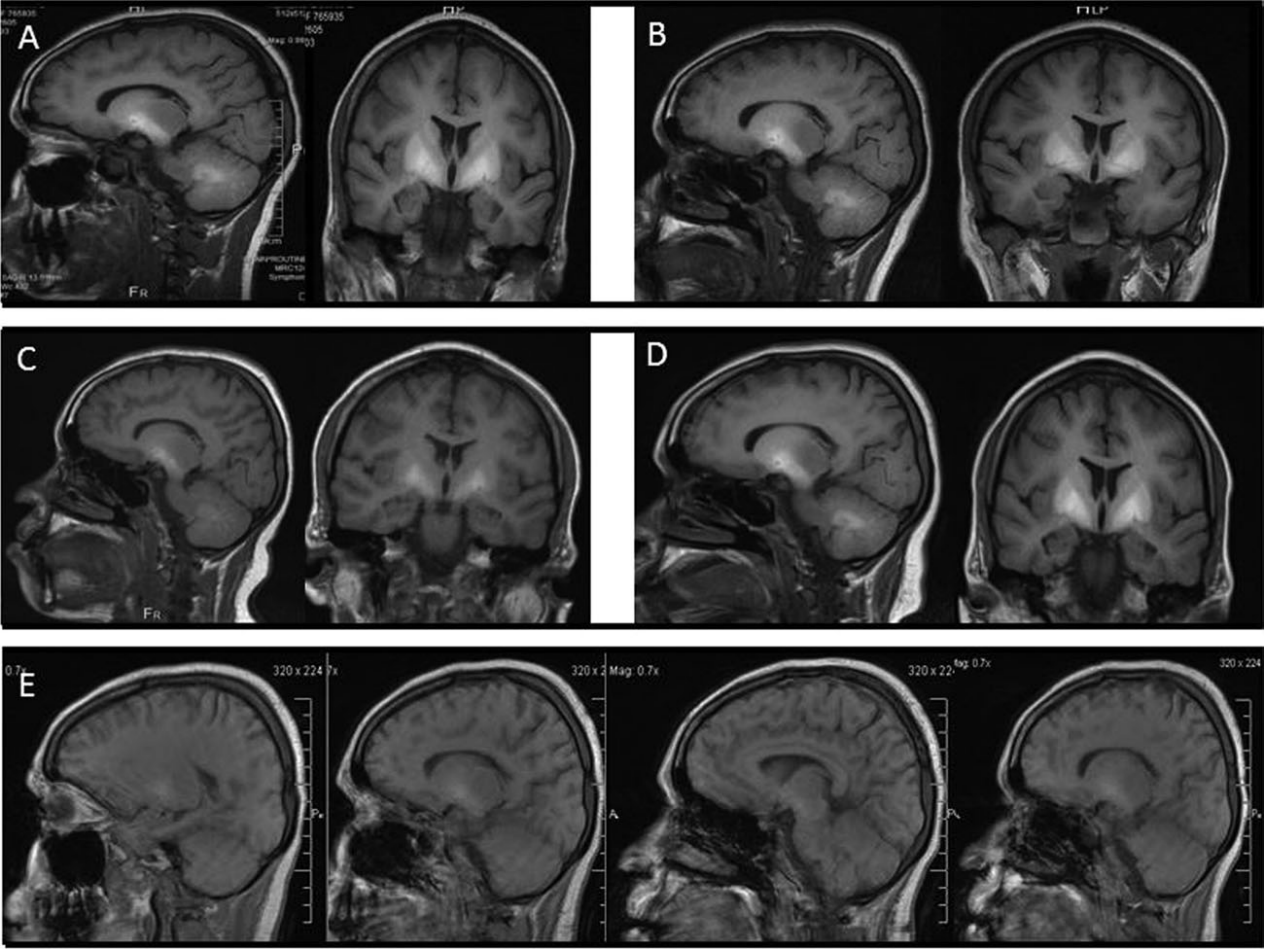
Serial MRI brain over a 10-year follow-up. (A) T1-sequences at the age of 12 (2002), before treatment. (B) T1-sequences at the age of 15 (2005), 3 years after treatment, slightly improved, compared to (A). (C) T1-sequences at the age of 16 (2006), 4 years under treatment, 1 year after increasing the frequency of infusions, and 1 month after adding oral ferrous iron, hyperintensities are less pronounced than before. (D) T1-sequences at the age of 20 (2010), 1 year after reducing chelation therapy, because of a lack of supplies. There is reaccumulation of manganese, compared to MRI 4 years earlier (C). (E) T1-sequences at the age of 21 (2011), 1 year after increasing the frequency of infusions, overall 9 years after onset of treatment, hyperintensities have clearly diminished.
